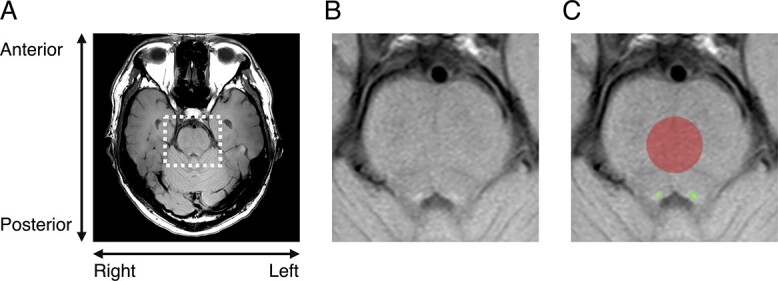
Individual examples of LC position. A) The whole image of a single participant measured by neuromelanin sensitive-weighted MRI. B) Enlarged view of brainstem area from (A). C) VOI of LC (green) and PT (red) superimposed on (B).
